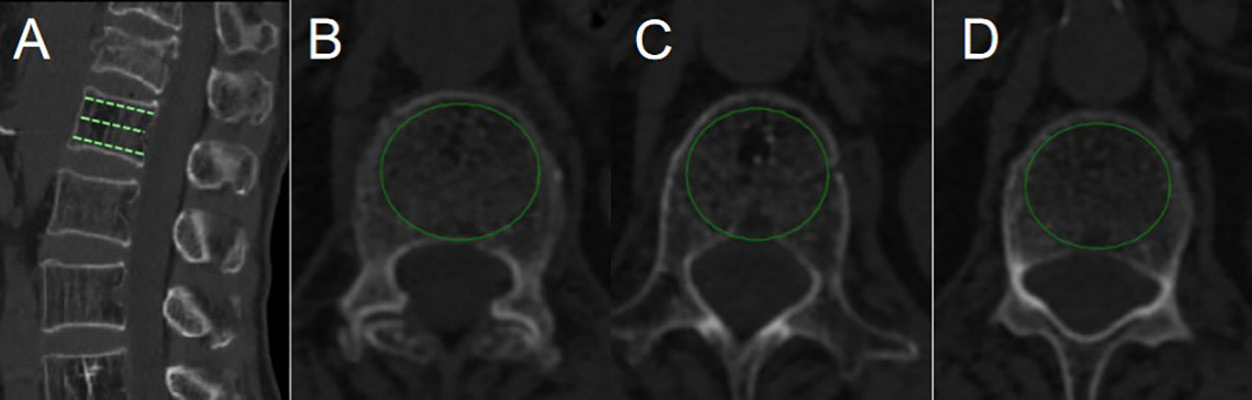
HU value was measured by PACS with the use of an elliptical region of interest function. (A) was a reconstructed CT sagittal image, marking the positions of the three slices. Slice (B) was chosen just inferior to the superior endplate. Slice (C) was taken in the middle of the body. Slice (D) was chosen just superior to the inferior endplate.
